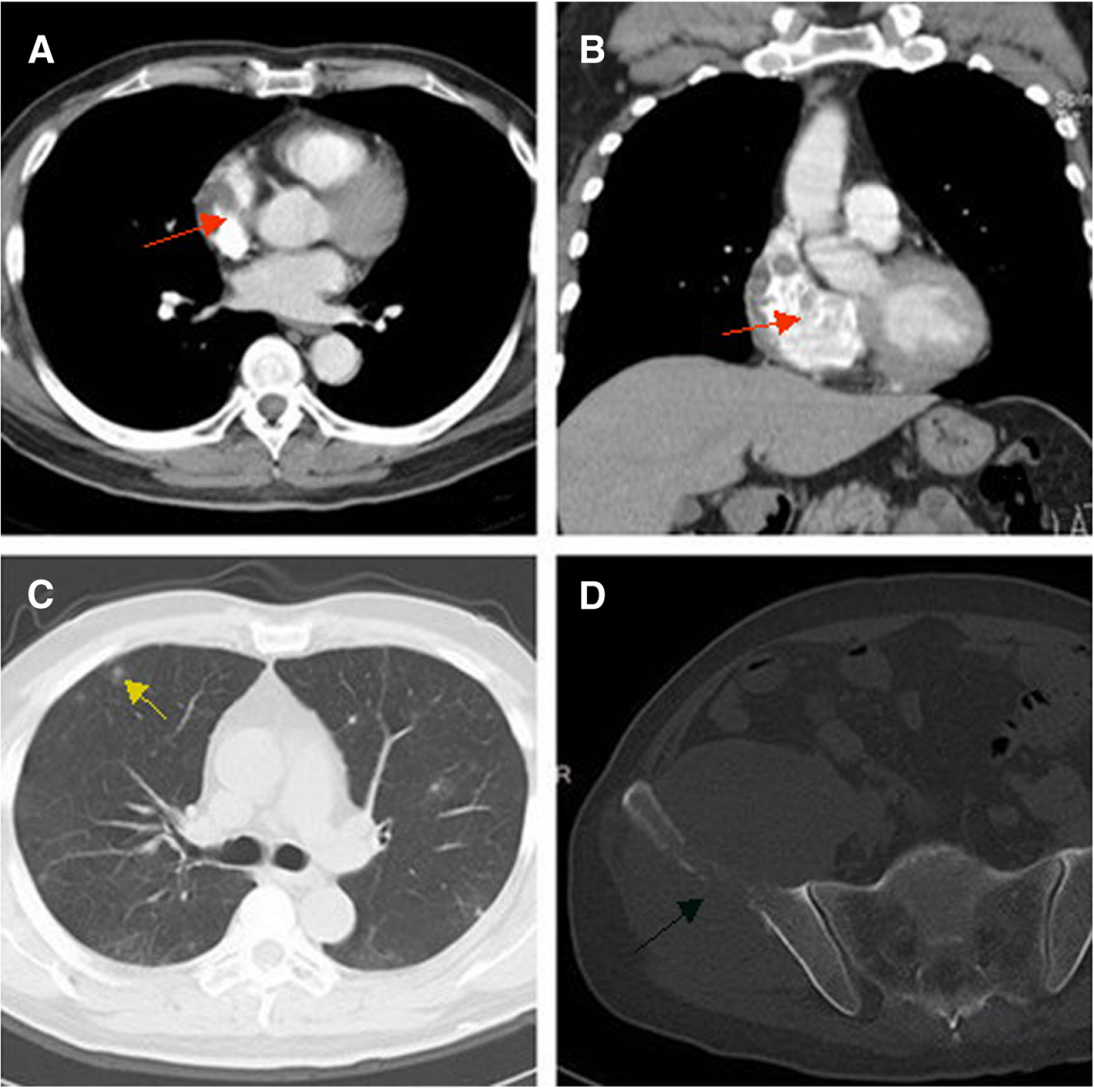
Male, 43 years old, right atrium angiosarcoma. a, b: Enhanced CT scans on both transverse sections and coronal sections indicate a mass with inhomogeneous enhancement (red arrows); c: Multiple metastatic nodules are seen in the bilateral lungs (yellow arrows), with ground-glass opacity in the nodule peripheries. d: The right ilium is involved (balck arrows), exhibiting osteolytic bone destruction, a blurry boundary, and a massive soft tissue mass
